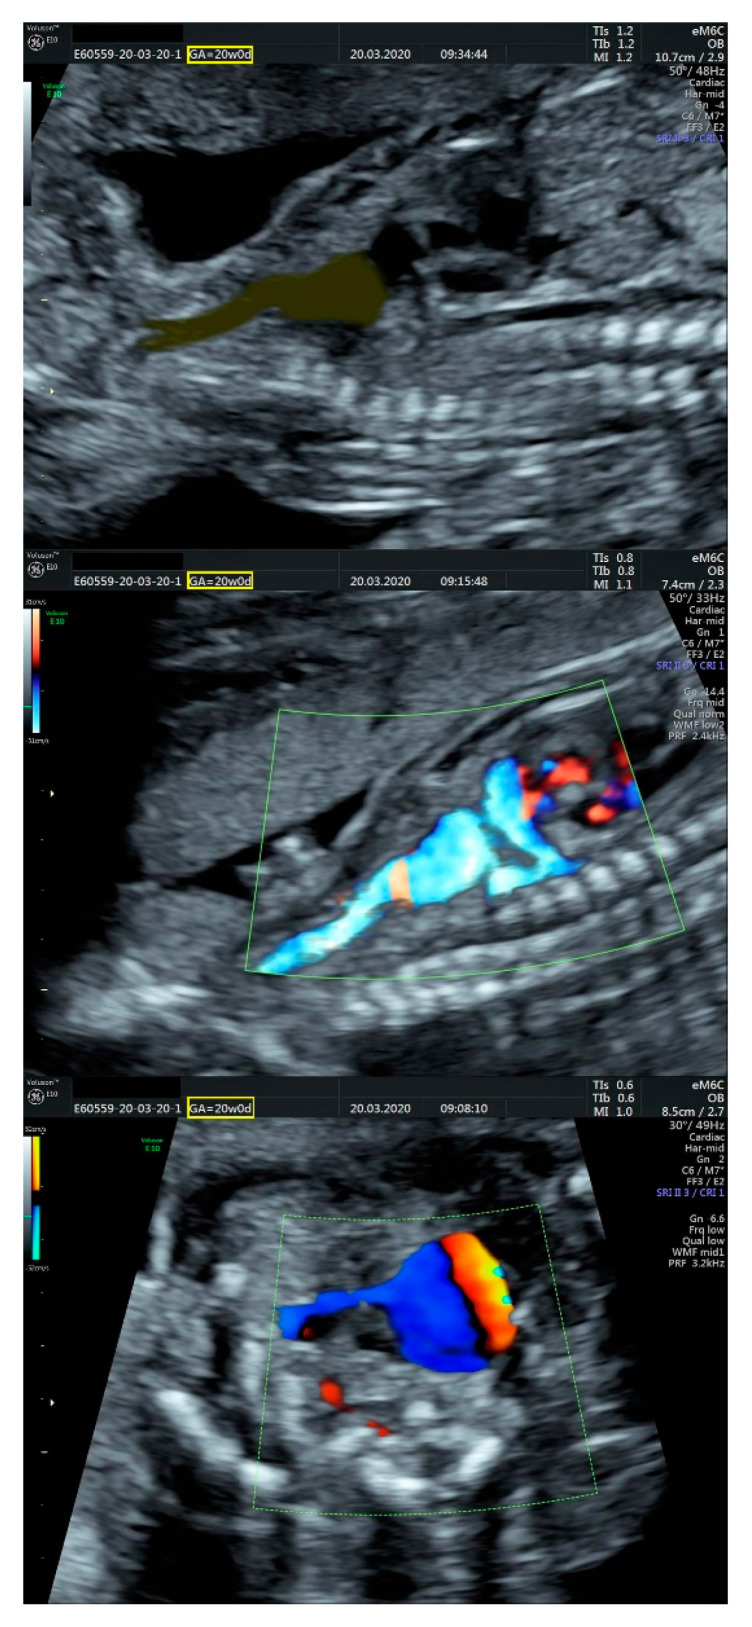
(Top and middle): sagittal section and Doppler examination of the aneurysm and the vascular pattern which bifurcates towards the cervical region; (bottom): turbulent Doppler flow highlighting the communication between the aneurysm and pulmonary trunk/left pulmonary artery.
